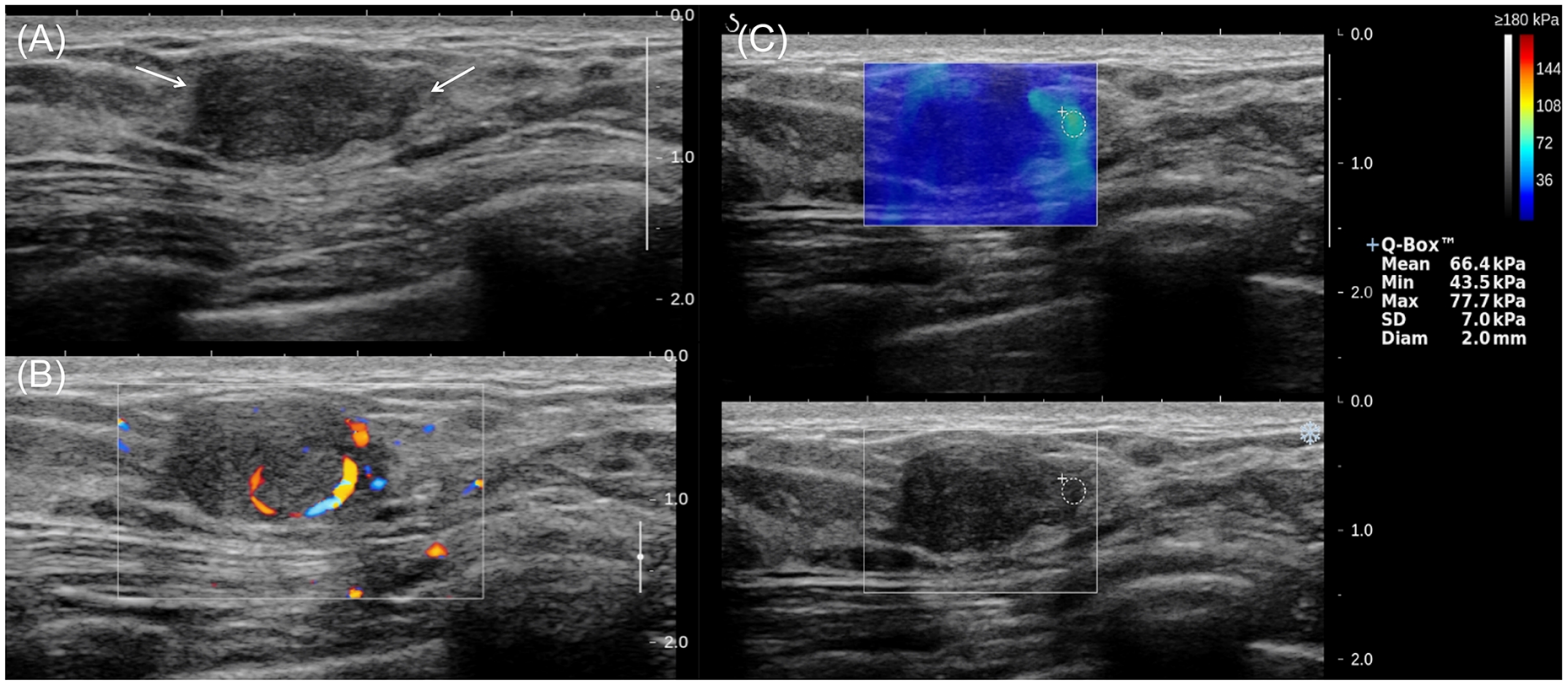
A 45-year-old woman diagnosed with phyllodes tumor by US-guided core needle biopsy, which was confirmed as benign phyllodes tumor on surgical excision. (a) The B-mode US image shows a 1.5-cm breast mass assessed as BI-RADS category 4a (arrows), (b) the color Doppler US image shows high vascularity, and (c) the shear-wave elastography image shows E_mean_ of 66.4kPa and E_max_ of 77.7kPa.
